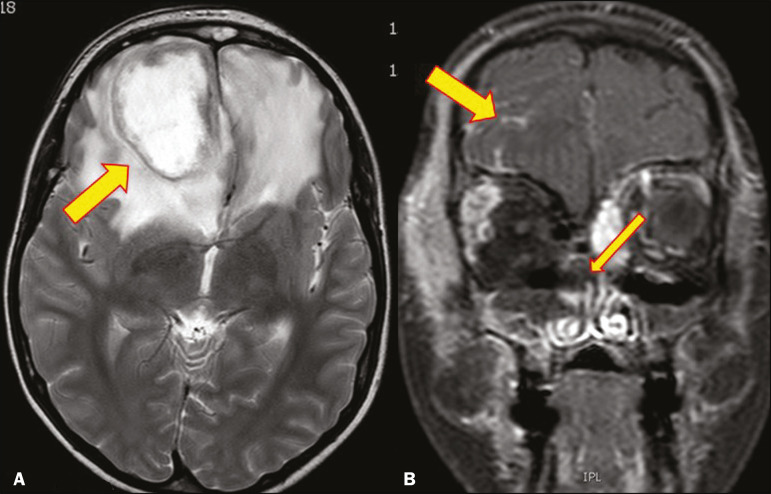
Mucormycosis with orbital and brain involvement. A: Axial T2-weighted MRI sequence showing a hyperintense lesion, a hypointense peripheral halo (arrow), and pronounced peripheral edema. B: Contrastenhanced coronal T1-weighted sequence showing a heterogeneous lesion in the right orbital region. Black turbinate sign (thin arrow), characterized by a lack of enhancement of the nasal turbinate mucosa. There is also leptomeningeal enhancement on the affected side (thick arrow).
